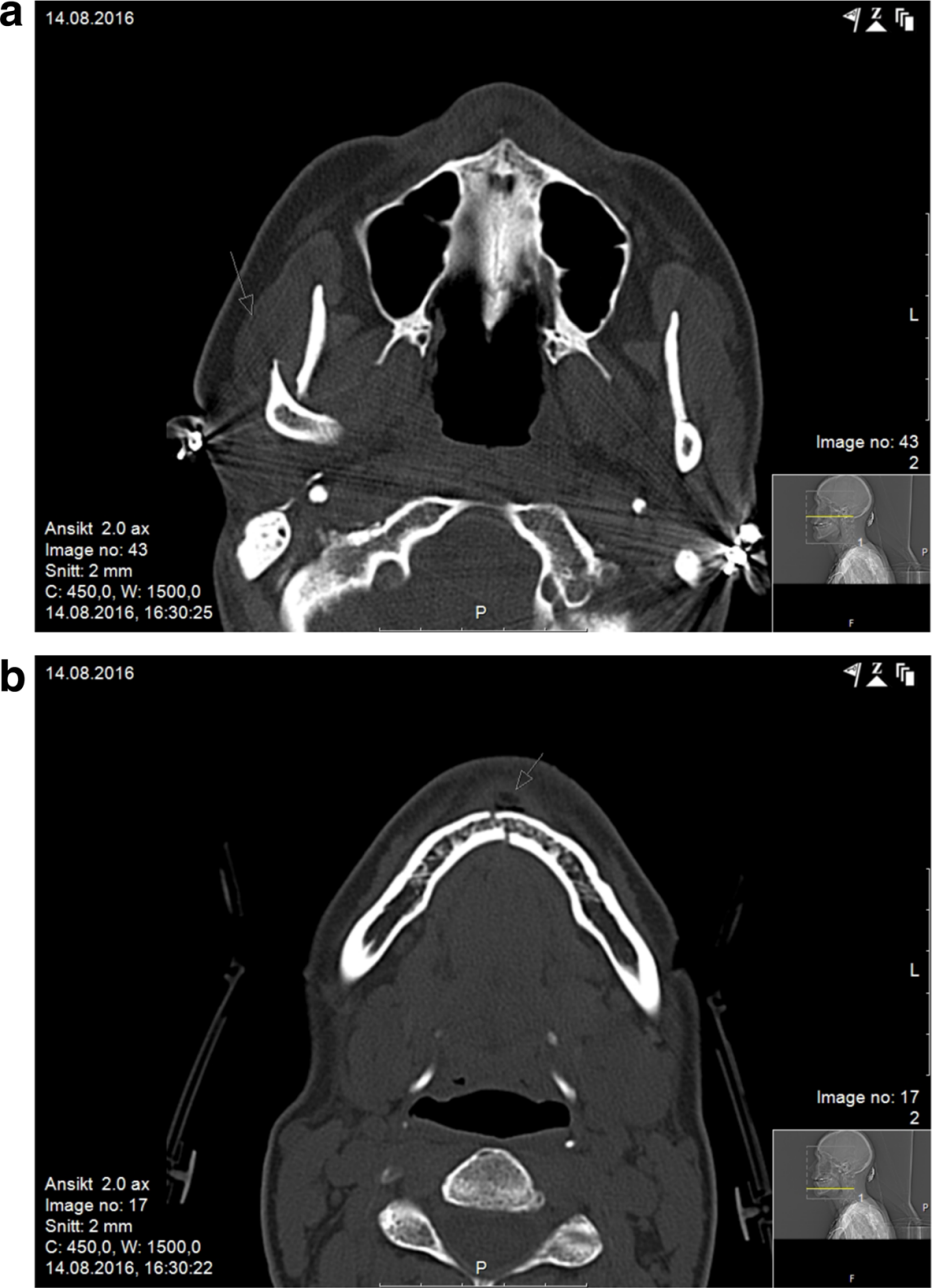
a, b Computed tomography scan at admittance shows fractures of the mandible (bilateral condylar neck and left corpus paramedian)
